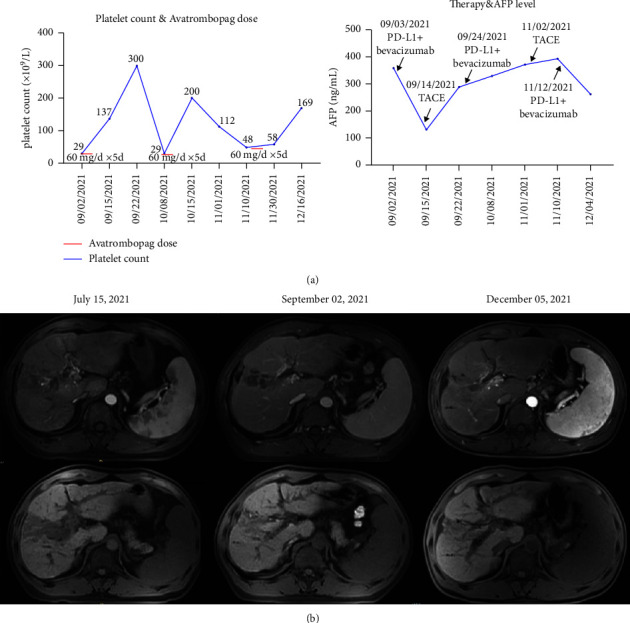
One typical case of the patient who successfully finished the scheduled anticancer treatment plan with the thrombopoietic effect of avatrombopag. (a) Platelet count, avatrombopag dose, the AFP level, and anticancer treatment over time; (b) changes in enhanced MRI of the patient with HCC.
